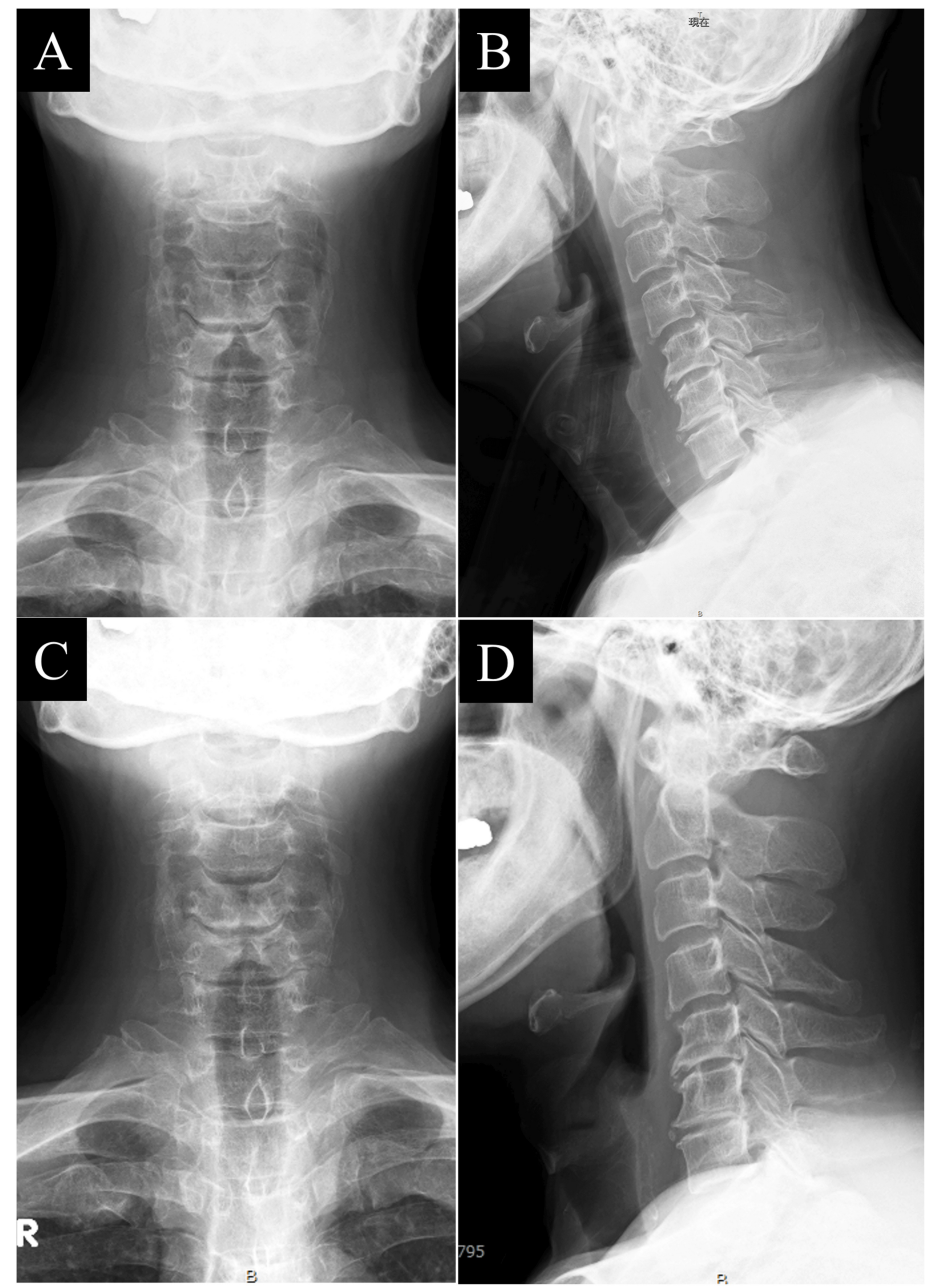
Plain radiographs of the cervical spine at the time of injury and six months post-injury. A: Plain radiograph at the time of injury. B: Plain radiograph at six months post-injury. No displacement of the fracture sites was observed from A to B.
